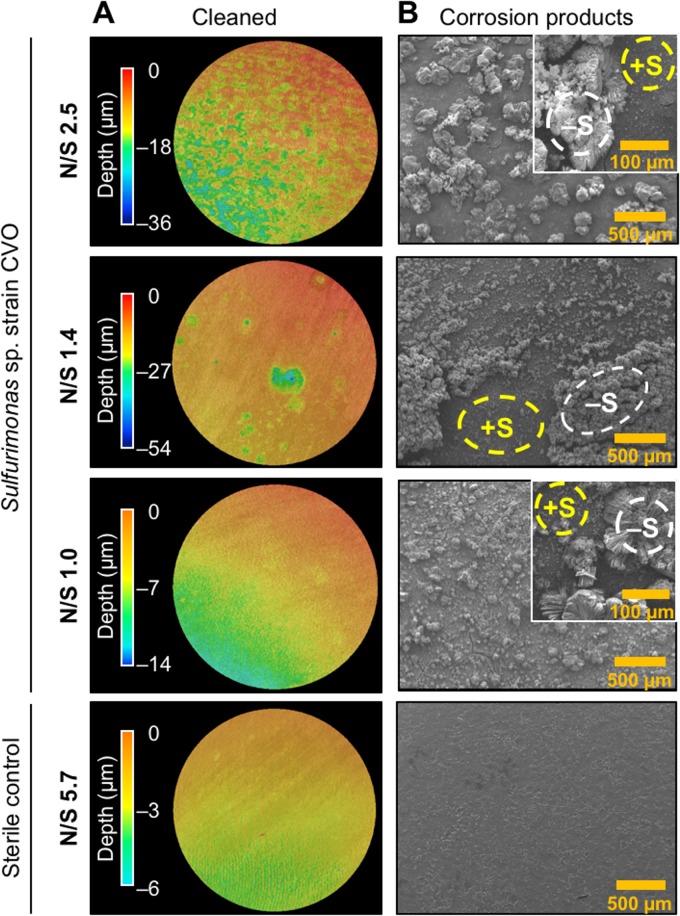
(A) Images obtained by white-light interferometry showing pitting corrosion of X52 carbon steel coupons (corrosion products removed). The color scale indicates depth of individual pits. (B) Energy-dispersive X-ray (EDX) microanalysis of corrosion crust deposited on the surface of carbon steel coupons used for weight loss corrosion analysis. High sulfur content (+S, yellow) was detected on the surface and was absent or markedly reduced in mineral structures (–S, white).
